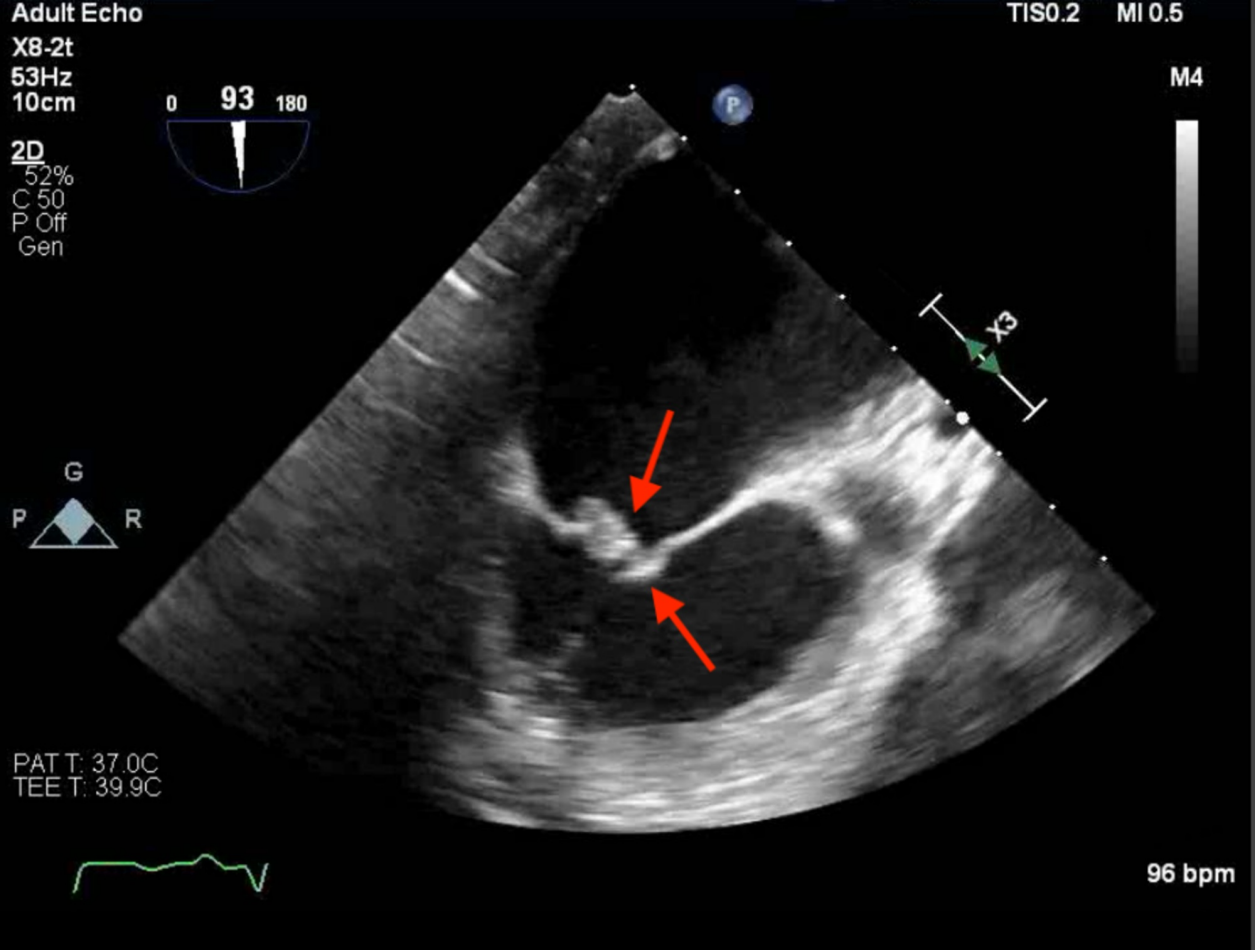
Transesophageal echocardiogram (TEE) The results of a TEE study: echogenic densities, approximately 0.6 cm in size, involving both anterior and posterior mitral leaflets, primarily at the tips, suggestive of endocarditis.
